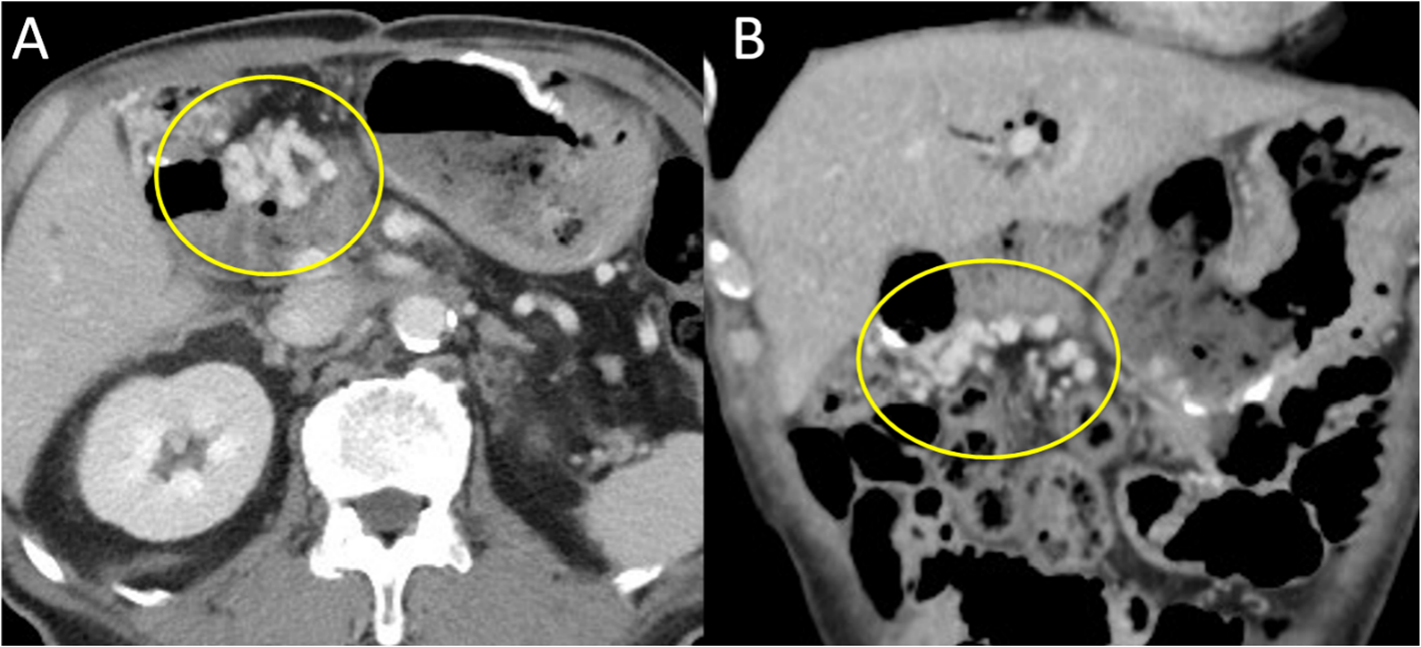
Findings of enhanced computed tomography (CT). a Axial imaging. b Coronal imaging. CT scan did not show obstruction or stenosis of the reconstructed PV, but it revealed transverse colonic varices (yellow circles)
